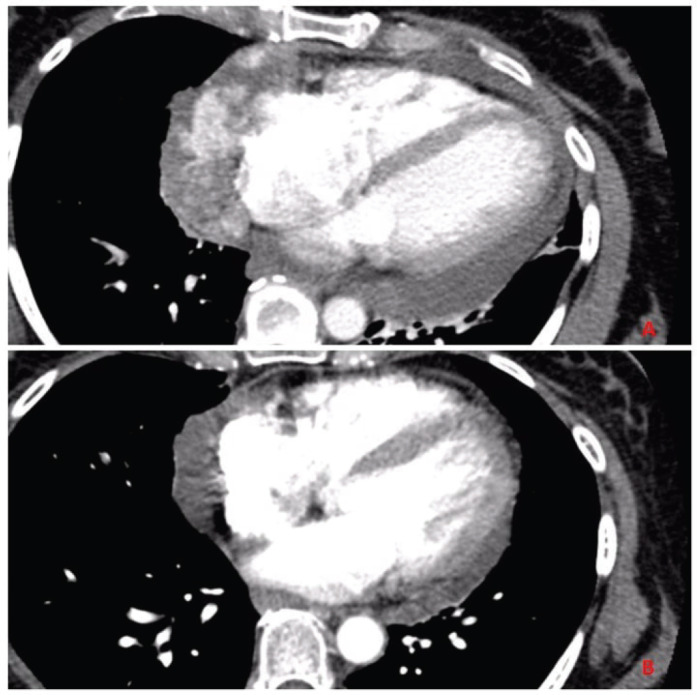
Panel A: CT chest with contrast before initiating chemotherapy shows invasive mass diffusely involving the pericardium and lateral right atrial wall. Panel B: CT chest with contrast after completion of chemotherapy showing partial response in the primary tumour.
